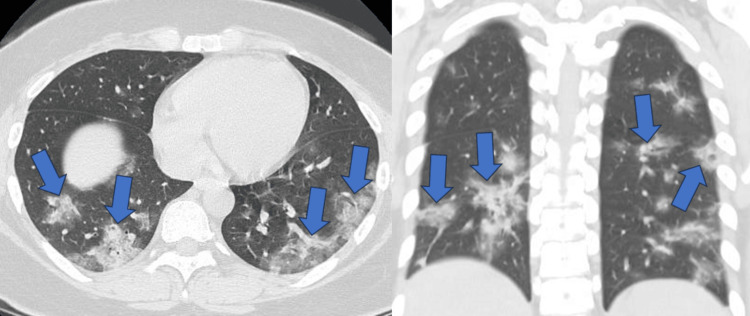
Patchy consolidation in bilateral lungs A female patient in her 20s was admitted to the hospital five days after the onset of symptoms of the alpha variant. Computed tomography (CT) revealed patchy consolidation in both lungs.
